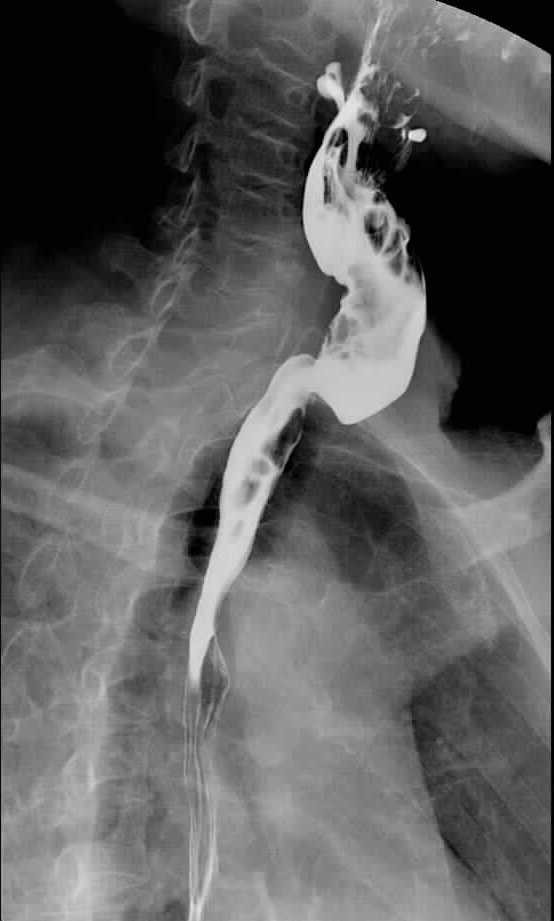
Barium radiography 6 months after the operation demonstrated that the reconstructed cervical esophagus was widely patent and smooth.
